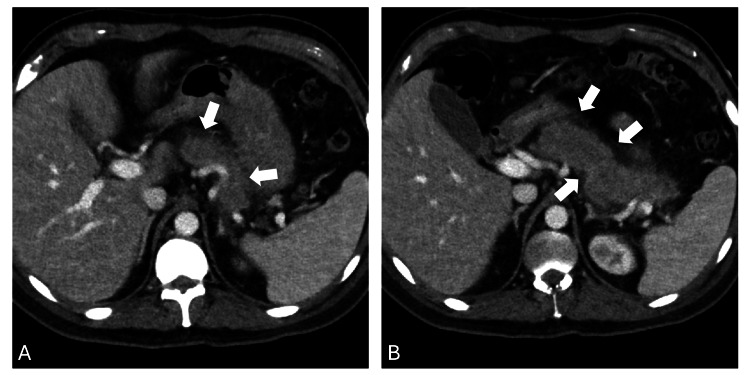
Edematous pancreas with peripancreatic fat stranding consistent with pancreatitis Panel A: Axial contrast-enhanced CT image showing an enlarged, edematous pancreas (arrow) with surrounding peripancreatic fat stranding, characteristic of acute interstitial pancreatitis. No evidence of necrosis or fluid collections is present. Panel B: Axial CT demonstrating peripancreatic fat stranding (arrow) and preserved pancreatic enhancement without ductal dilatation or pseudocyst formation, consistent with uncomplicated acute pancreatitis.
